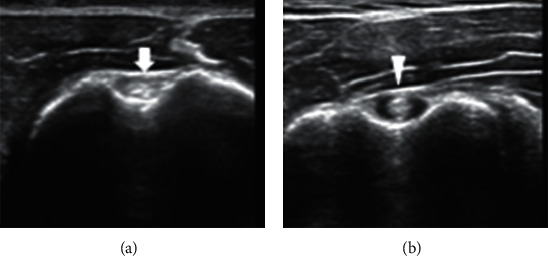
Ultrasound images of LHB tendon in healthy (a) and painful (b) shoulders. LHB tendon in healthy and nonpainful shoulder (arrow). LHB tendon in painful shoulder (arrowhead). The tendon was rounded and enlarged, with effusion in the tendon sheath.
